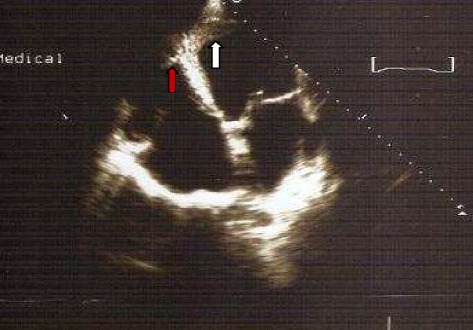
Apical four chamber view showing near complete dissolution of right ventricular thrombus (red arrow) and marked decrease in left ventricular thrombus (white arrow) after six months of anticoagulation.
